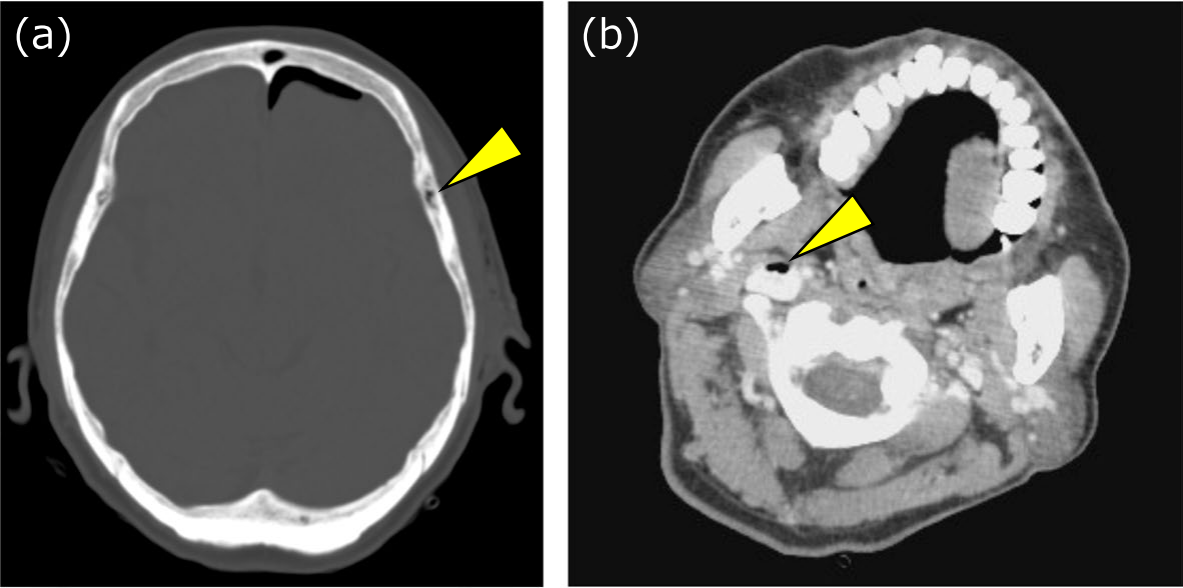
Postoperative CT findings in case 2. a CT brain image showing air densities in the diploic layer of the skull. b CT brain image showing air densities in the right internal jugular vein
